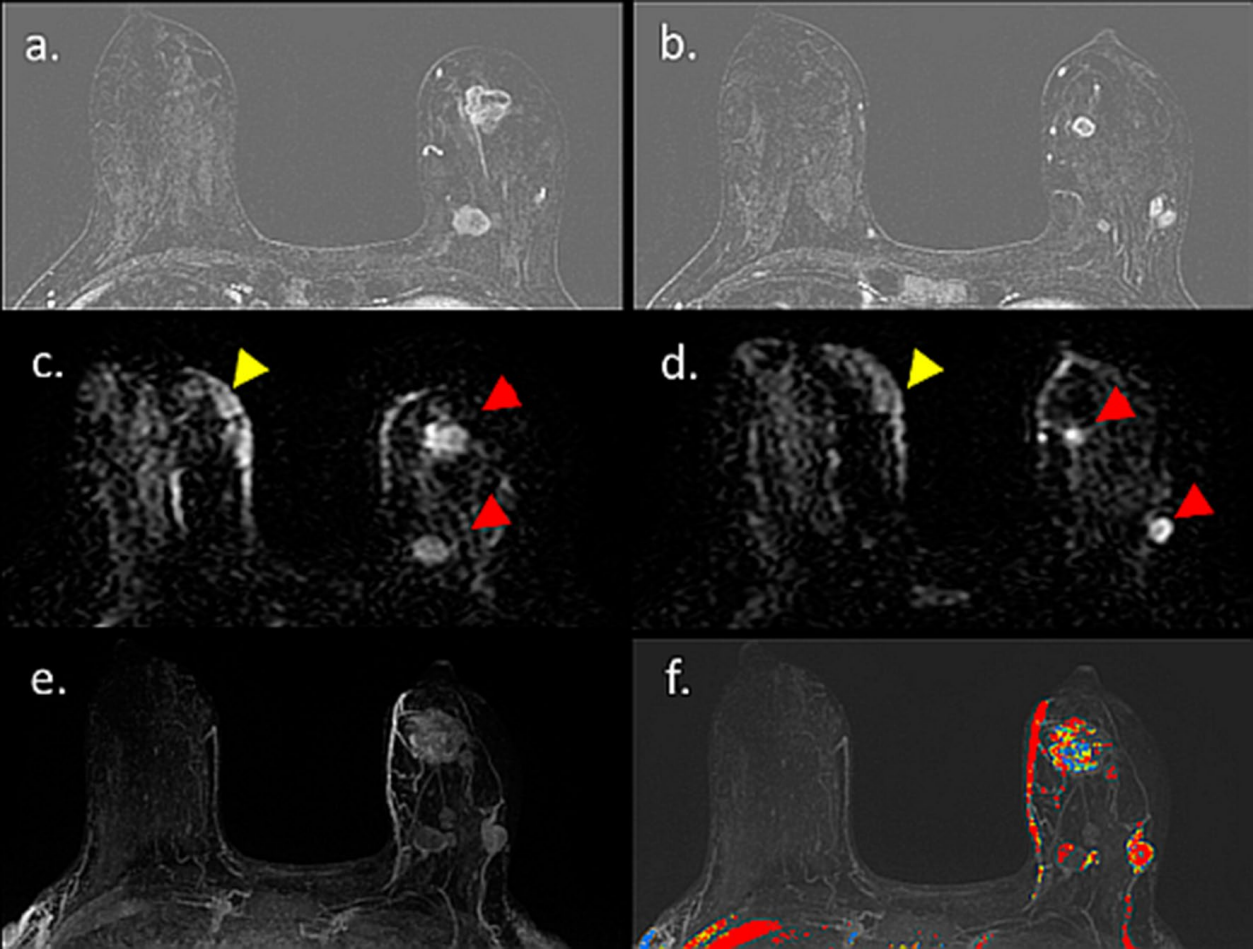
MRI of multi-centric IDC during lactation. MRI of a 40-year-old PABC patient (lactating for 18 months) is presented. The woman presented with a palpable left breast mass and was referred to pre-operative MRI upon IDC diagnosis. *Note*: Several multifocal tumor foci are shown on the subtracted DCE MRI of the left breast (red arrow heads), surrounded by a moderate BPE (grade 2) (a, b). In agreement, diffusion weighted images (b = 700) revealed restricted tumor regions (c, d), though incomplete fat saturation artifacts are also presented on the right breast (yellow arrow heads). The entire multifocal tumor distribution could be further appreciated on MIP images of DCE and CAD (e and f, respectively)
